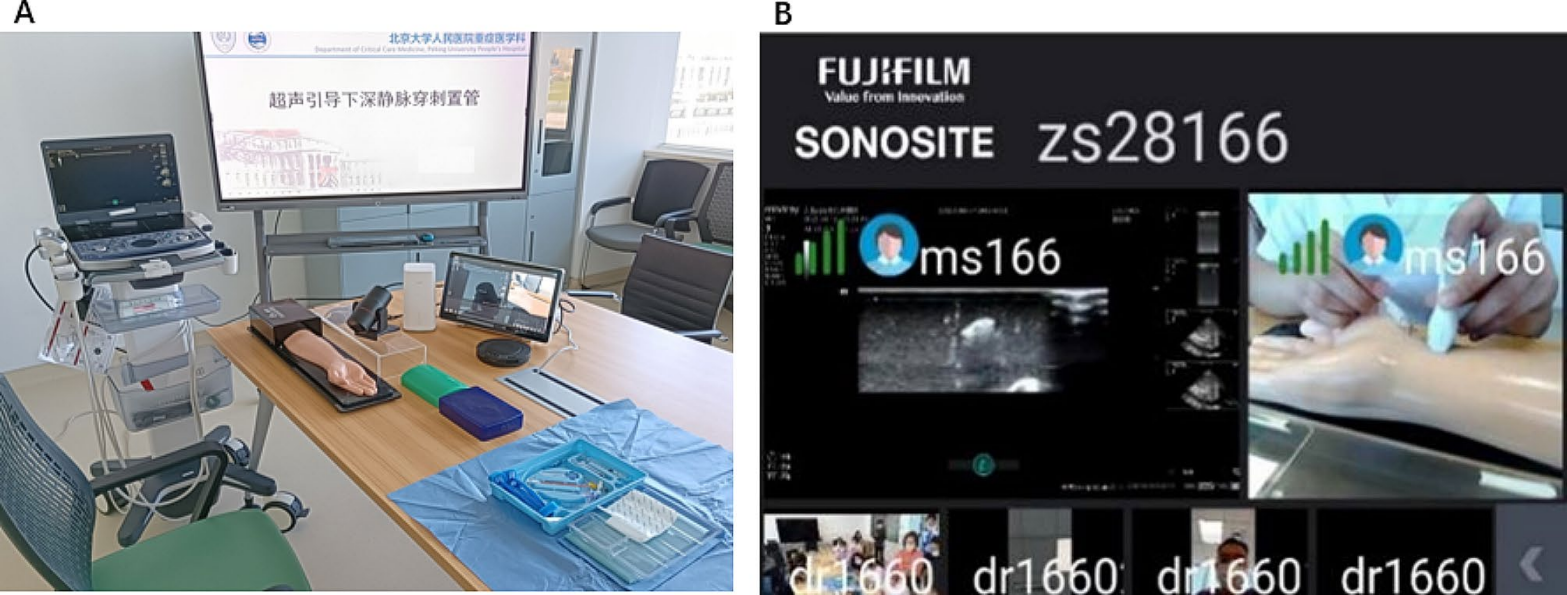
Interactive 5G remote critical care ultrasound training device. A: 5G remote critical care ultrasound visualization cloud platform device connection; B: 5G interconnected multi-platform operator image transmission and debugging
